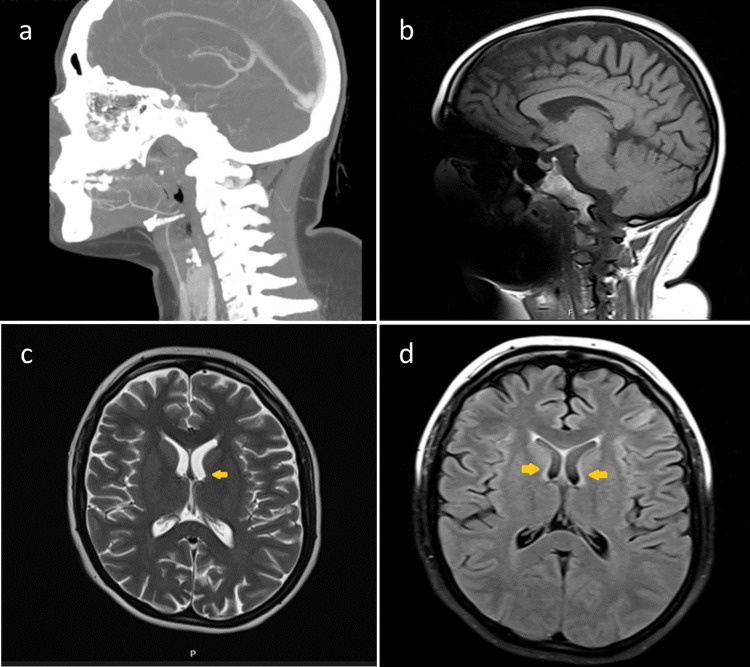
Neuroimaging of a patient with thiamine deficiency. Sagittal CT (a) showing no significant bony abnormalities; sagittal T1-weighted MRI (b) with preserved brain anatomy; axial T2-weighted MRI (c) showing symmetric hyperintensities in the dorsomedial thalami; axial FLAIR MRI (d) demonstrating hyperintensities in the dorsomedial thalami and periaqueductal region, consistent with thiamine deficiency.
